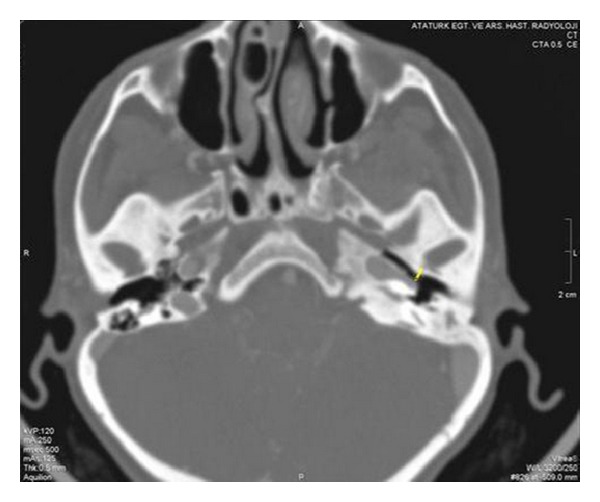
Axial CT scan of the temporal bone showing the aberrant carotid artery, entering the tympanic cavity through a dehiscent carotid plate.
